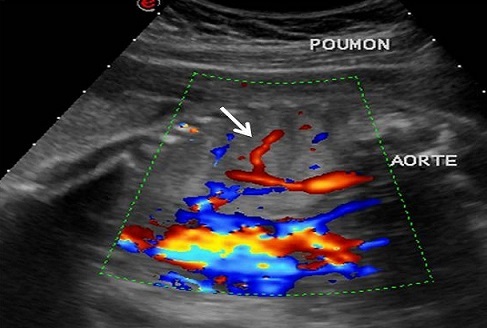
Left sagittal sonogram of fetal chest: echogenic pulmonary mass and visualisation of systemic feeding artery arising from the abdominal aorta (arraw)
